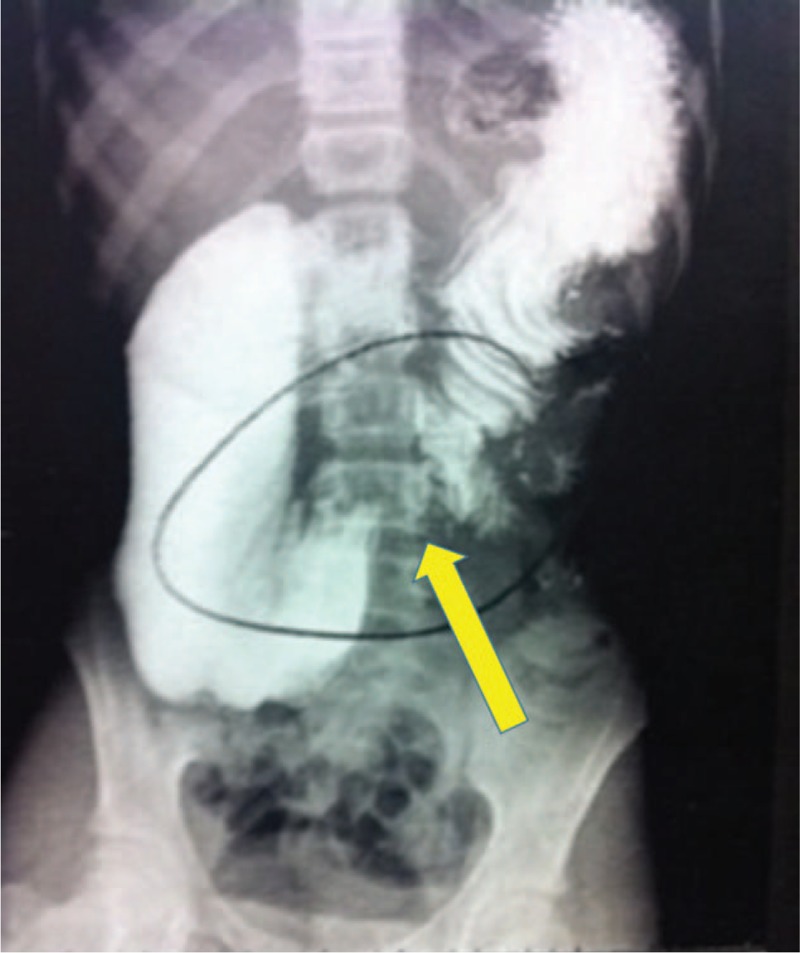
Contrasted abdominal x-ray showing duodenal dilatation with a progression stop of the contrast in the transition between 3^rd^ and 4^th^ duodenal portions.
